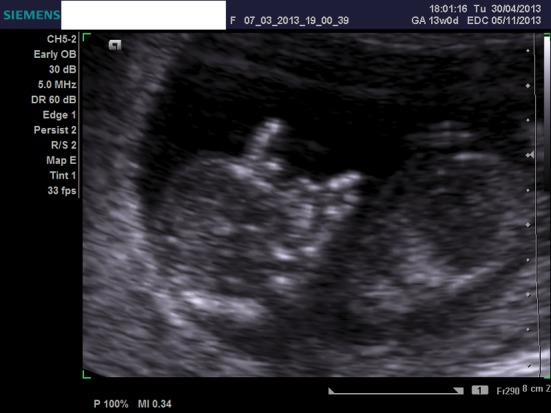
Ultrasound image of the second fetus at 12 weeks, showing the nuchal translucency and present nasal bone.
